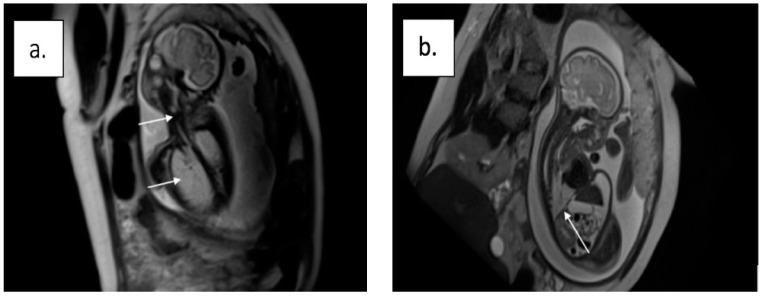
Fetal magnetic resonance image at 30 weeks of gestation: (a) airway discontinuity, hyperechogenic lung, and (b) flattened diaphragm.
